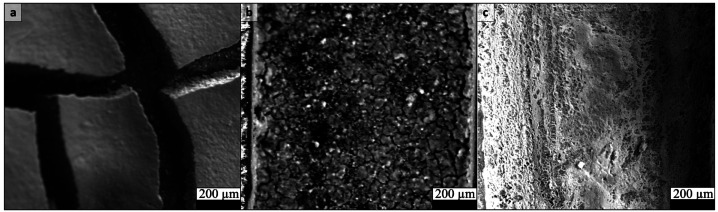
SEM images of drop-casted thin films of TiO_2_ with different mixing ratios and dispersion methods. (a) a 1:1 (wt:wt) TiO_2_–IPA mixture with no ultrasonication. (b) a 1:10 (wt:wt) TiO_2_–IPA mixture with no ultrasonication. (c) a 1:10 (wt:wt) TiO_2_–IPA mixture with 10 min of ultrasonication.
